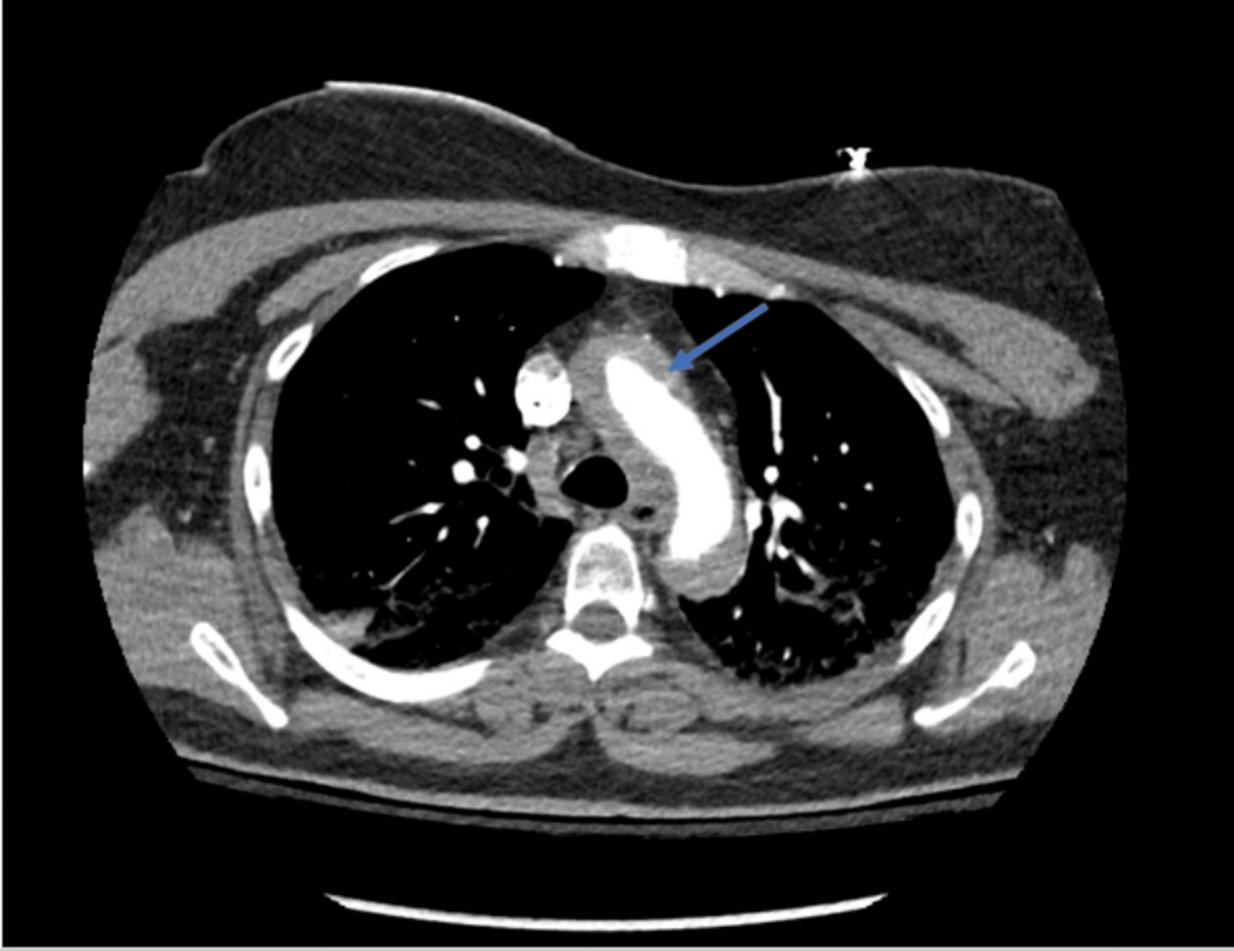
CTA of the chest (axial view) with and without IV contrast showing acute type A Stanford dissection (arrow) involving the ascending and descending aorta CTA: CT angiography
